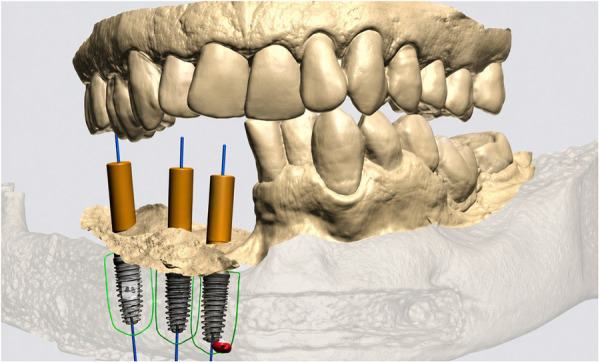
CBCT imaging and prosthetically driven digital implant planning following fibular free-flap reconstruction.
